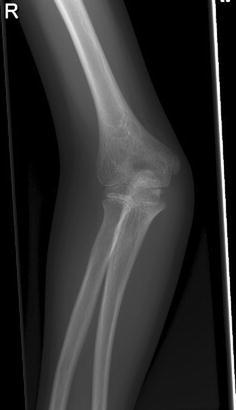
Anteroposterior (AP) radiograph of the elbow. Note the valgus angle and the dislocation of the radial head
